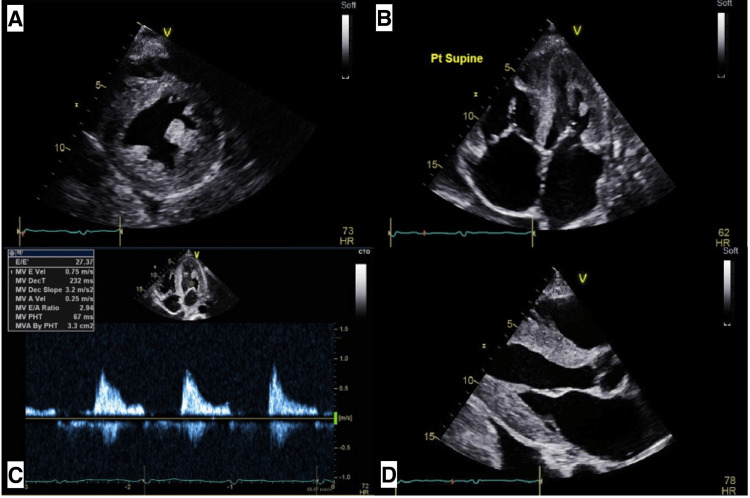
Transthoracic echocardiography. (A) Thickened LV on short axis view. (B) Bilateral atrial enlargement on apical view. (C) Grade 3 diastolic dysfunction. (D) LA dilation and LV hypertrophy on long axis view. LV: left ventricle; LA: Left atrial.
